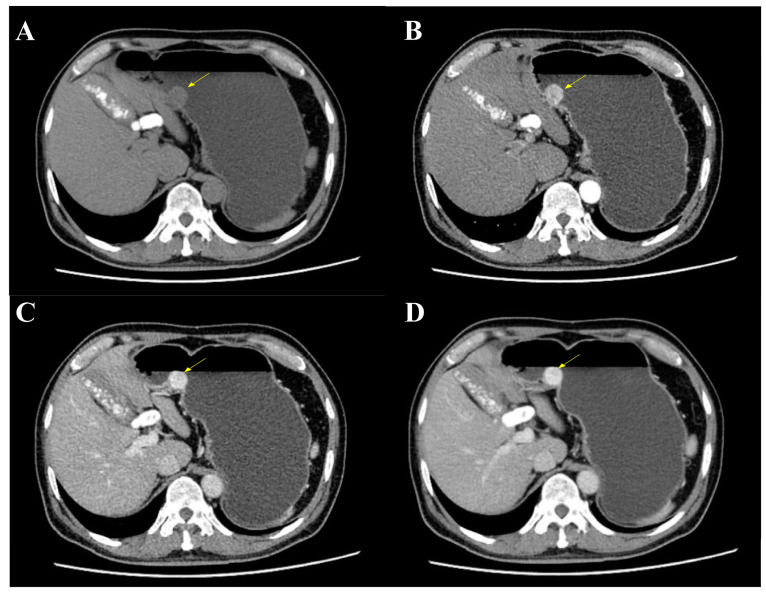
Abdominal and pelvic enhanced CT. (A) CT showed a mass at the gastric body with a clear boundary and soft tissue density (yellow arrow). (B) The mass was enhanced during arterial phase (yellow arrow). (C) An increased enhancement of the mass was found during portal venous phase with a maximum CT value of 156HU (yellow arrow). (D) The mass was still enhanced during delayed phase (yellow arrow).
